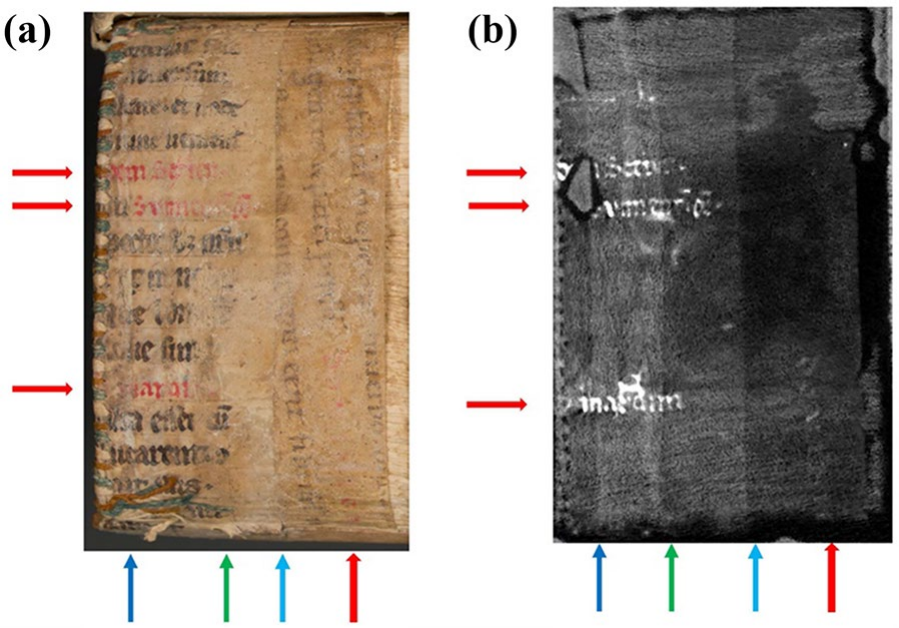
(detail of Fig. 2b). Comparison of parchment images taken by digital camera (a) and CT scan processed with LOGISMOS (b). In both (a) and (b), the horizontal and vertical red arrows point to the location of red lettering; in (b), the dark blue line points to the edge of the underturned portion of the first strip, the green line points to the edge of the second strip where it extends underneath the first, and the turquoise blue line points to the right edge of the first strip
